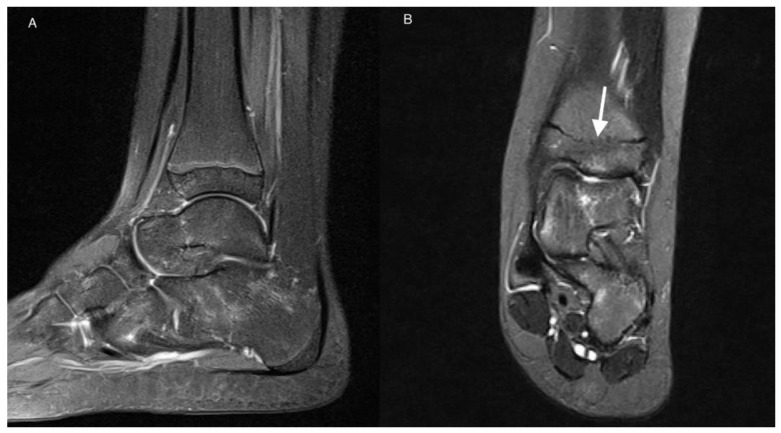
MRI of the left ankle of an 11-year-old girl with JIA. T2-weighted TIRM images in sagittal (A) and coronal (B) planes. Patchy BME (stage 1) in talus and calcaneus. Developmental disorder in the form of premature closure of physis (arrow).
